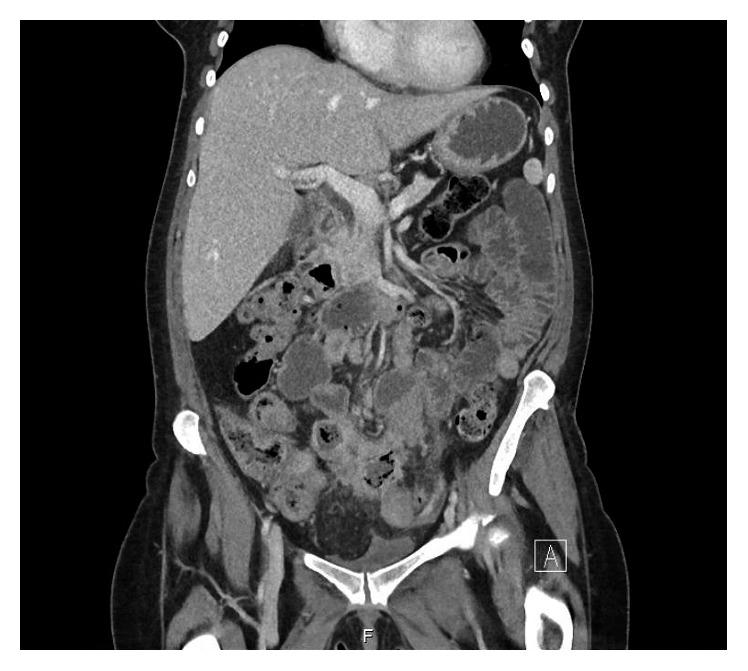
CT scan with unspecific inflammatory changes.
